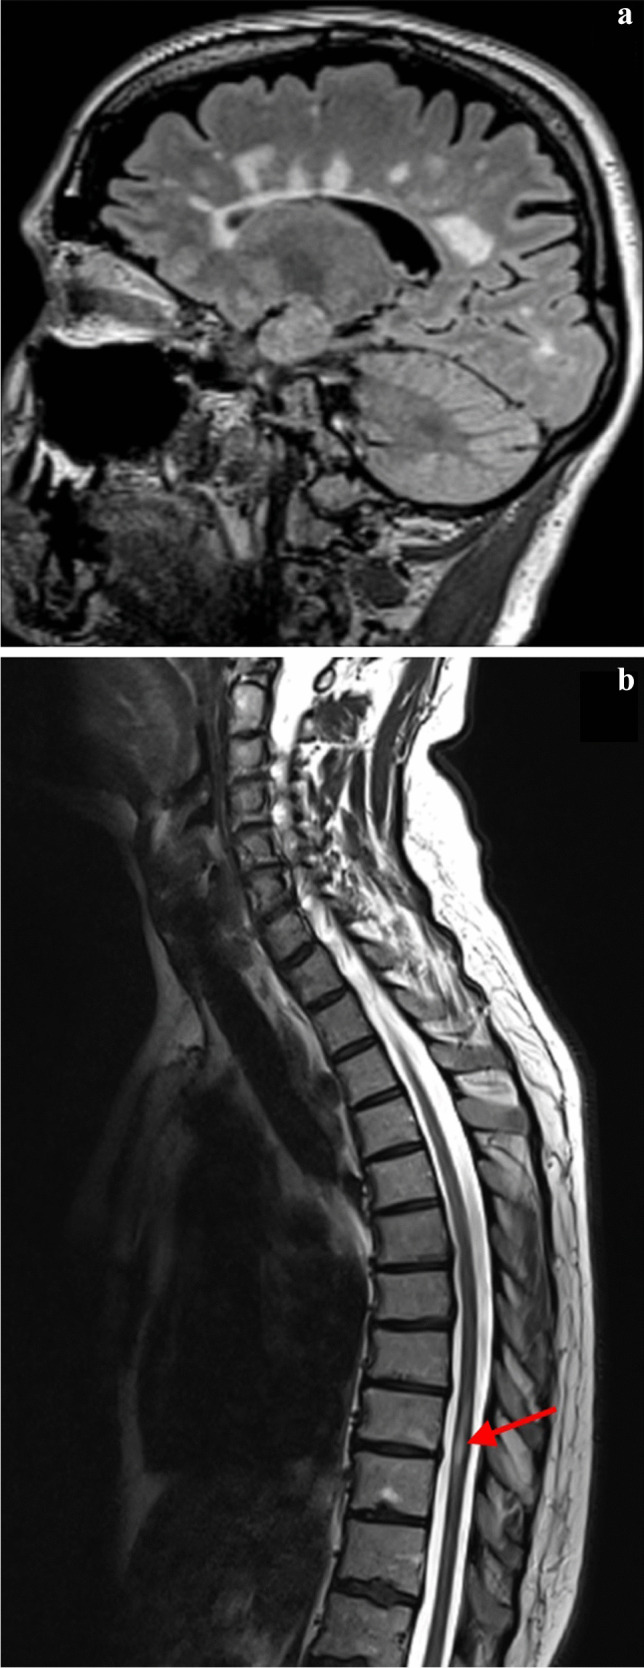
MRI of the patient 1.0. Sagittal FLAIR shows callososeptal hyperintensities radiating from the lateral ventricles with a typical perpendicular orientation (a). Sagittal T2 weighted image shows typical intramedullary lesion (b)
